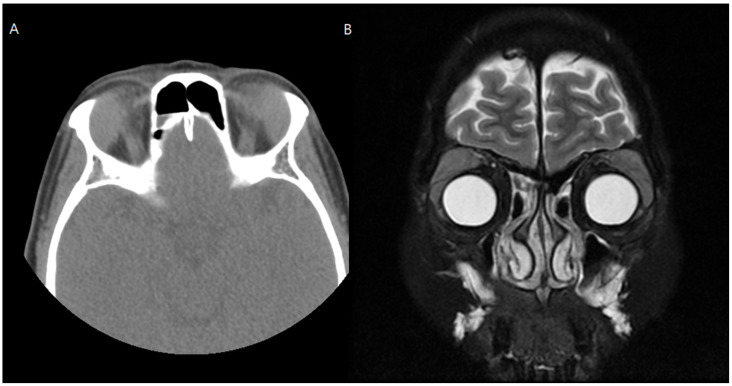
Preoperative computed tomography (A) and magnetic resonance imaging (B) of Case 2. Enlarged lacrimal glands were noted in both orbits.
